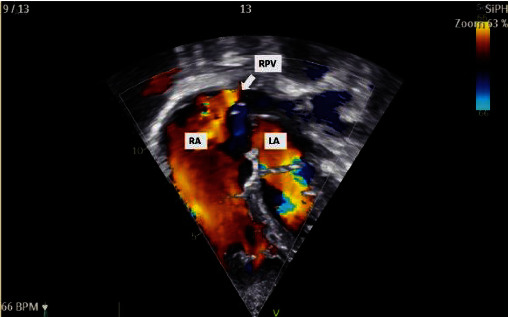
Echocardiography showing a four-chamber view with partial anomalous right pulmonary venous drainage with blood flow from the right lower pulmonary vein to inferior vena cava. LA: left atrium; RA: right atrium; RPV: right pulmonary vein.
